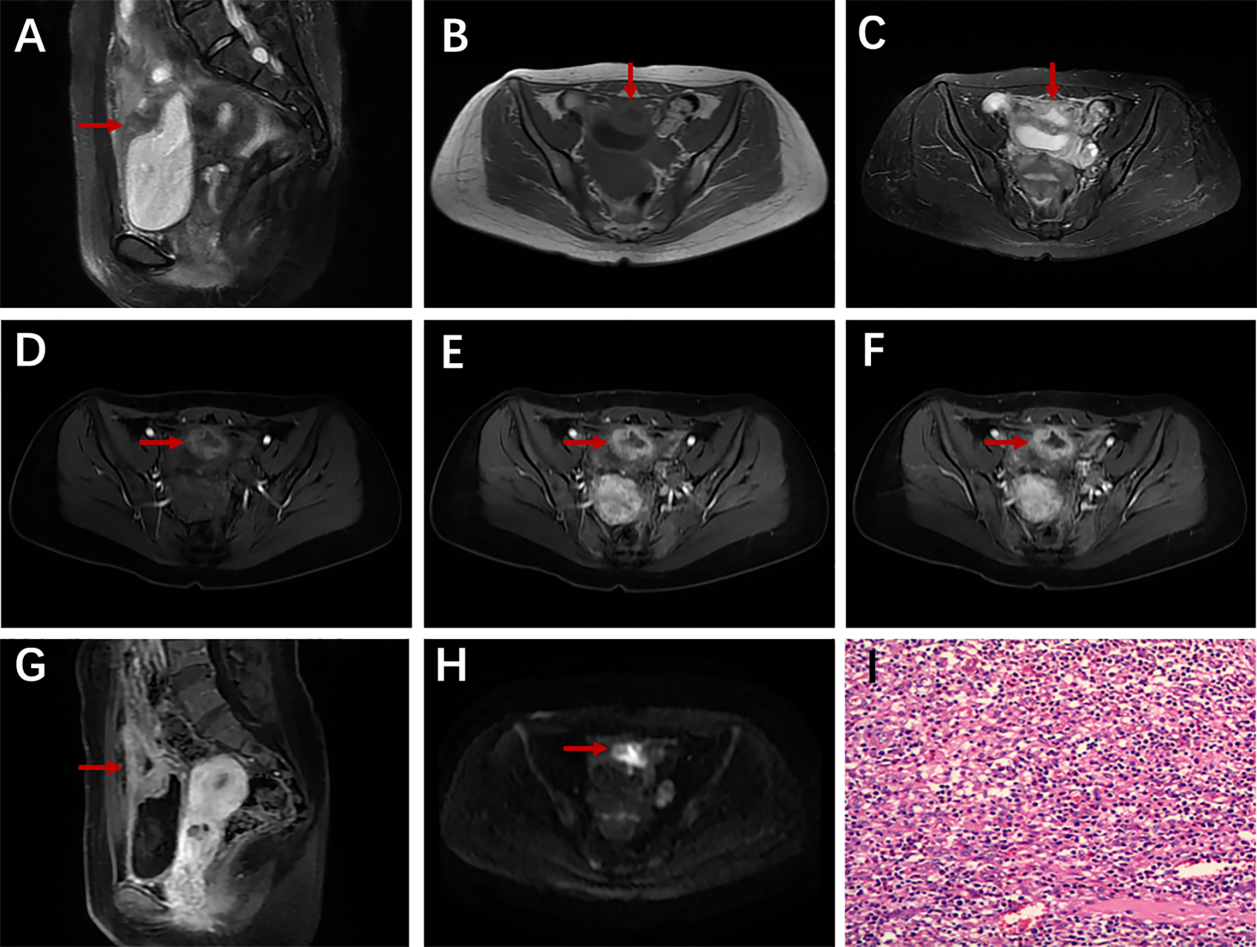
A 32-year-old woman with urachal cyst and infection presented with pollakiuria. Sagittal T2WI (A) showed a heterogeneous mass at the junction of the urachus and bladder; the cyst wall showed iso-intensity on T1WI (B) and slightly hyperintensity on T2WI (C); axial dynamic contrast-enhanced LAVA-Flex images showed apparent enhancement of the cyst wall (D–G); mildly restricted diffusion in the cyst wall on DWI (H); Pathology showed a large number of acute and chronic inflammatory cell infiltration (I).
